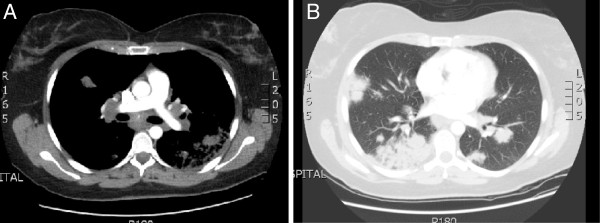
Computed tomography scan of thorax. (a) Extensive mediastinal and bilateral hilar lymphadenopathy. (b) Multifocal patchy air-space consolidation in both lungs.
